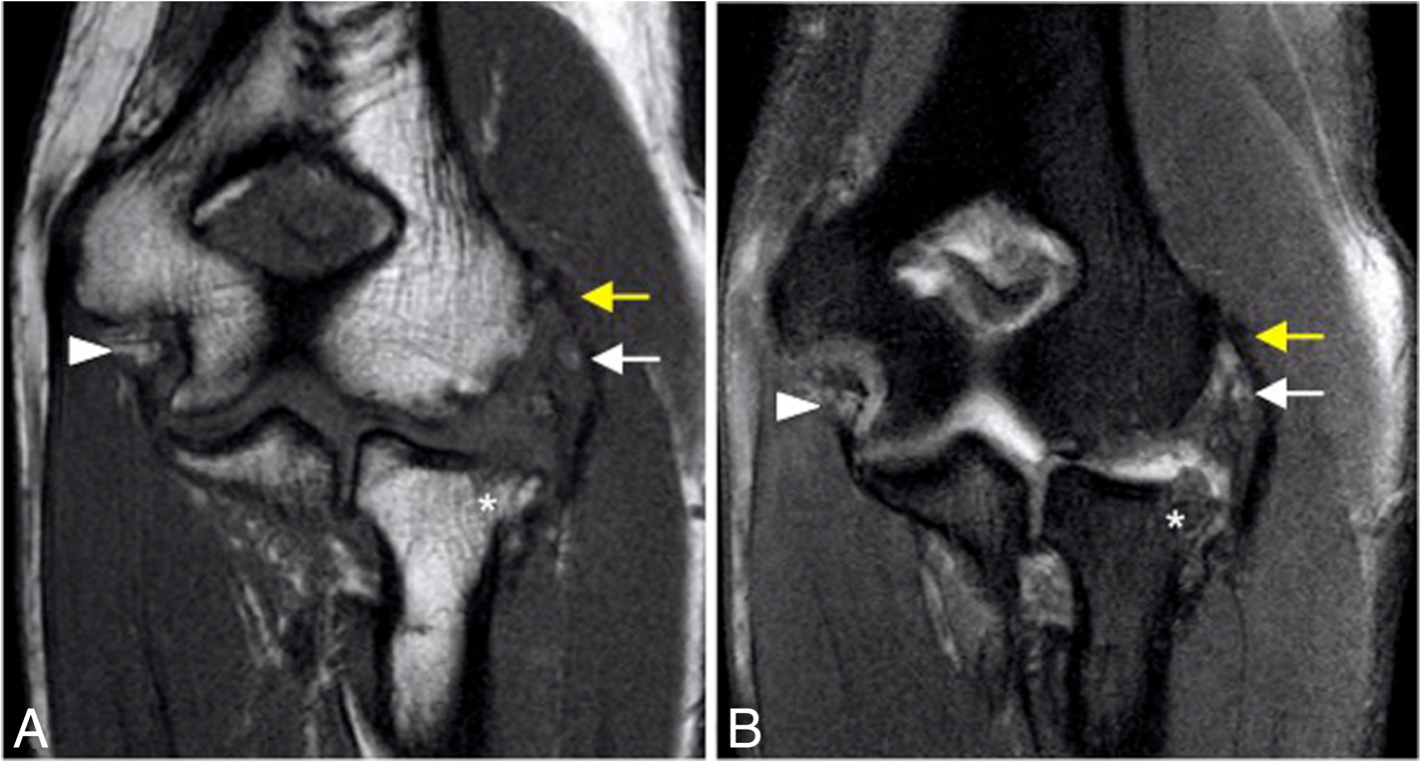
Posterolateral rotatory instability, stage 3B. Coronal T1-weighted MRI (a) and coronal FS PD-weighted MRI (b) showing an acute proximal common avulsion of the lateral ulnar collateral ligament and radial collateral ligament (white arrows), an acute avulsion of the anterior bundle of the medial collateral ligament complex (white arrowheads), a radial head fracture (white asterisks), a partial tear of the proximal common extensor tendon (yellow arrows), and joint effusion
